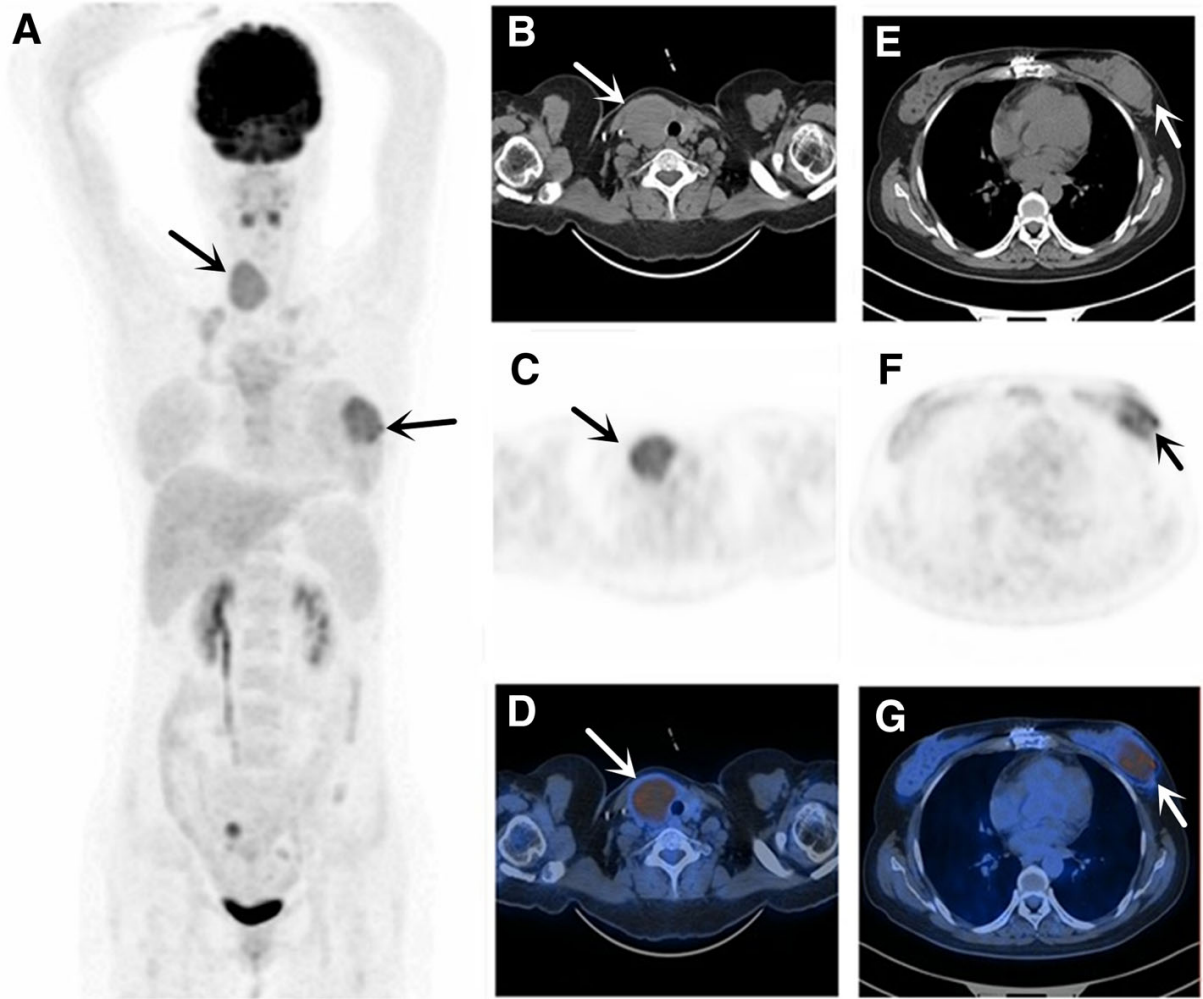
Case 12, a 38-year-old woman found to have a mass in the right thyroid, and ultrasound-guided fine-needle aspirations (USG-FNA) revealed EMP of the thyroid. Without evidence of systemic spread according to other clinical examination, suspicion of EMP was made. ^18^F-FDG PET/CT was performed for further evaluation. Maximum intensity projection (a), CT, PET and fused PET/CT images (b-g) showed extensive hypermetabolic lesions involving the right thyroid, and an additional lesion in the left breast (surgery confirmed EMP) was also identified. Due to detection of new lesions in the breast, more invasive therapy was given, including enlarged surgical region, enlarged field irradiation and systemic therapy, instead of resection of thyroid plasmacytoma combined with RT alone
